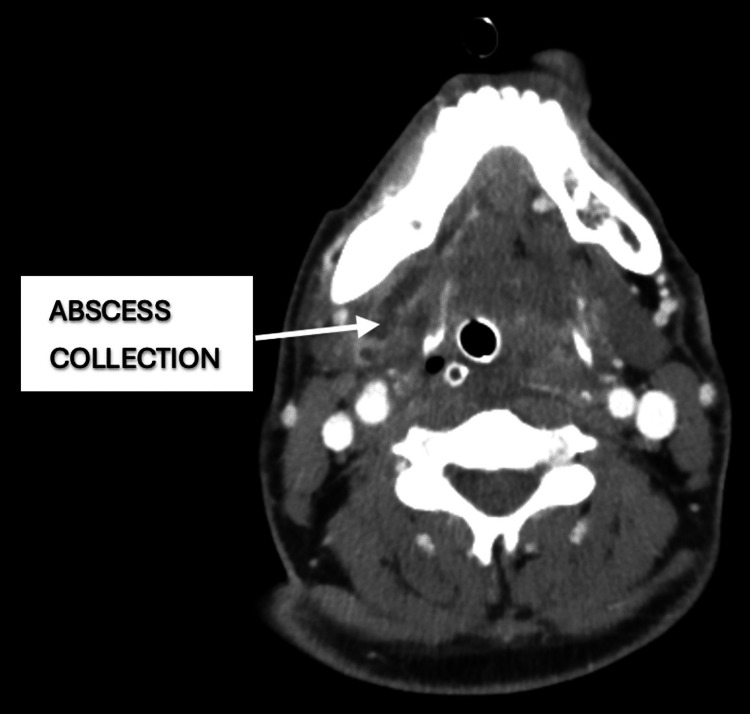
CT of the neck showing the 4.5 cm abscess in the submandibular-submental space. CT: computed tomography
